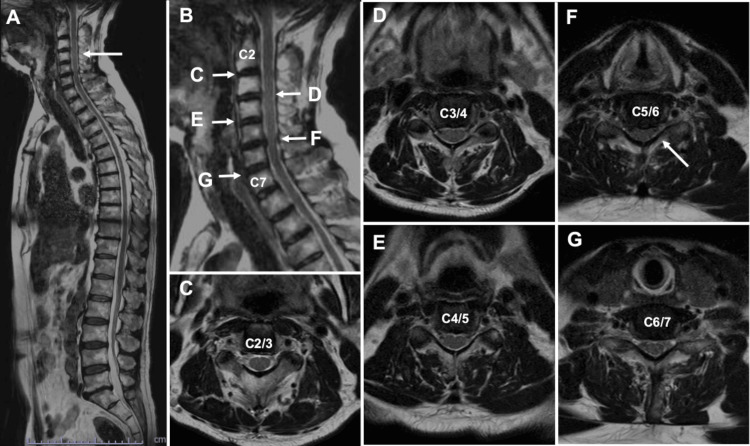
Initial whole spinal magnetic resonance images (A, B) Cervical degenerative spinal stenosis (white arrow) was observed, but no lesions were identified in the thoracic and lumbar levels. Hyperintensity lesions in the spinal cord were not observed, either. (C-G) The cervical spinal canal stenosis was observed from C2 to C6. The spinal canal was narrow, especially at the level of C5/6 (white arrow in F).
